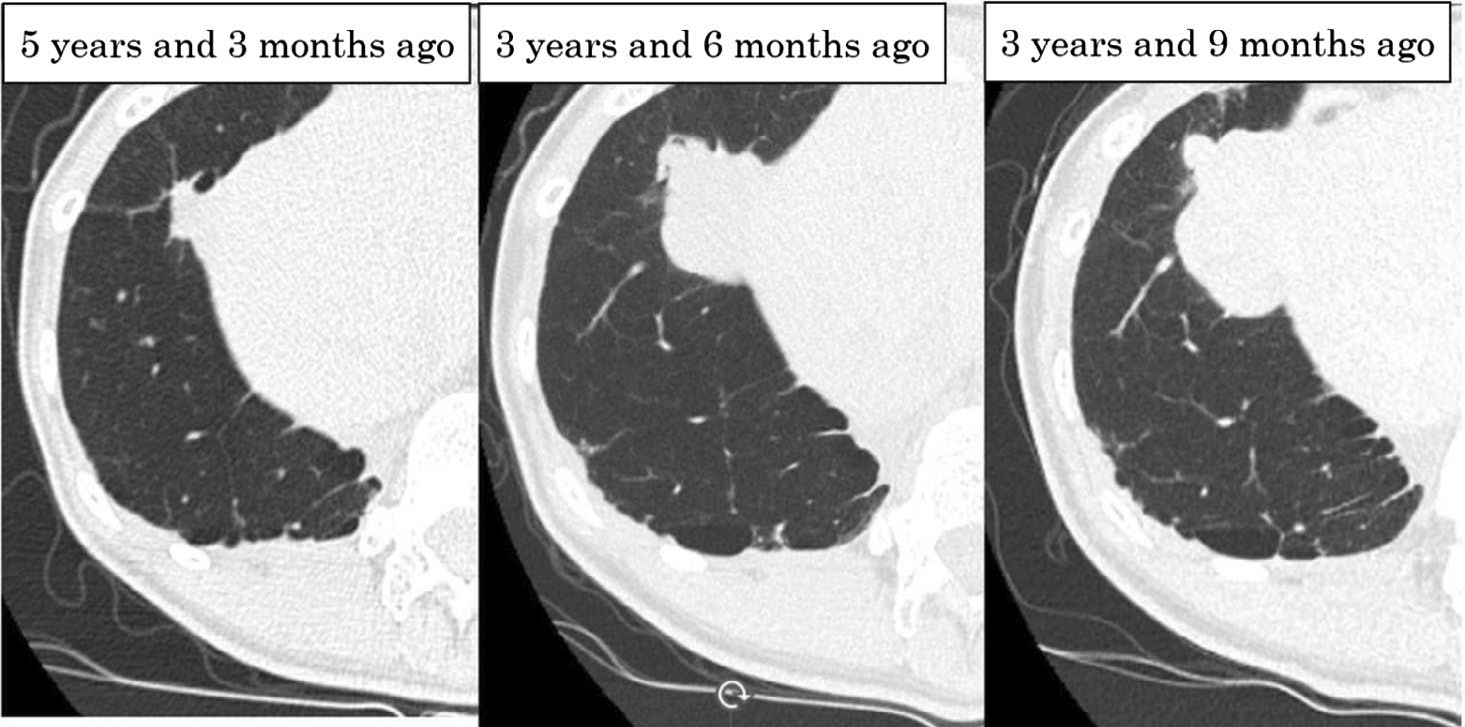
Computed tomography scan showing a nodule in the right middle lobe. The nodule increased in size to 4.5 cm over 18 months.
